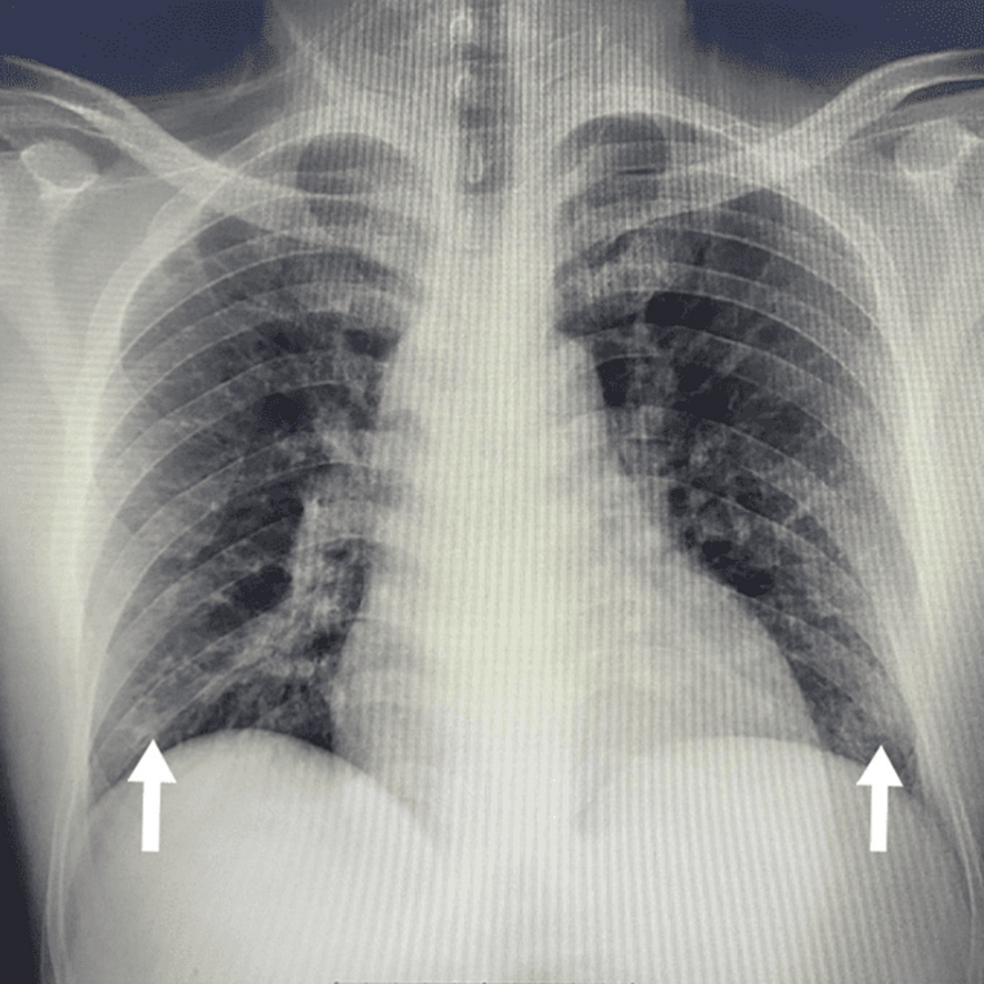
Chest X-ray showing bilateral infiltrations (white arrows)
